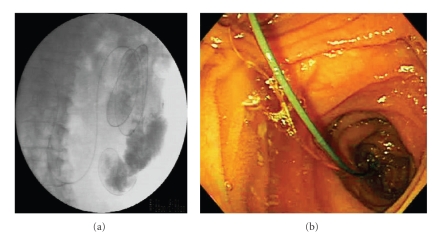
Rendezvous technique for acute angulation of Roux-en-Y limb. (a) X-ray film showed that a guidewire advanced over the Roux-en-Y limb. (b) Endoscopic imaging revealed that a guidewire reached the Roux-en-Y limb.
